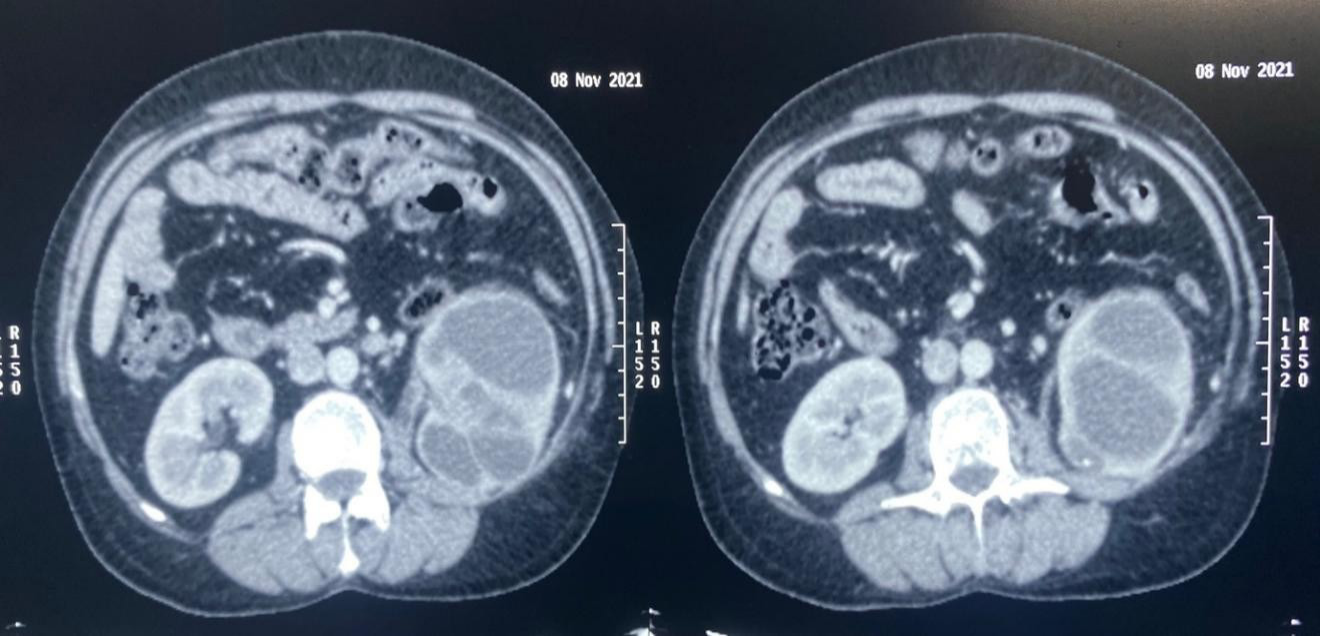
First computed tomography urography.
